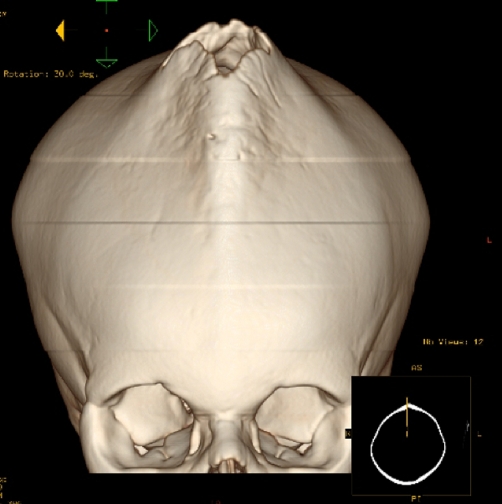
CT Brain showing bony sclerosis and thickening of the skull base due to osteopetrosis.
